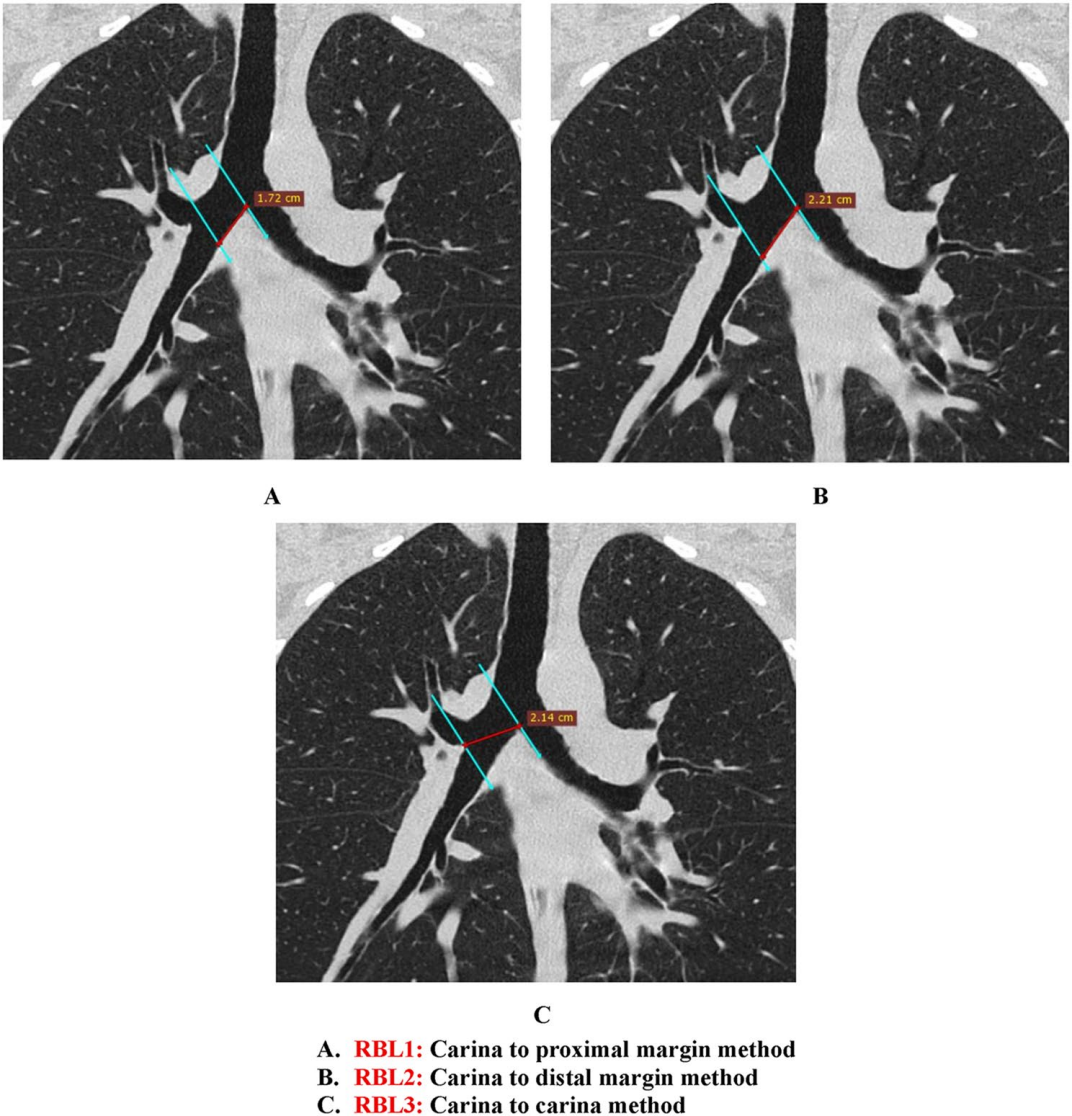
Coronal chest CT reconstruction (lung window) demonstrating right main stem bronchus length measurements using three methods. A. RBL1: Carina to proximal margin method. B. RBL2: Carina to distal margin method. C. RBL3: Carina to carina method
